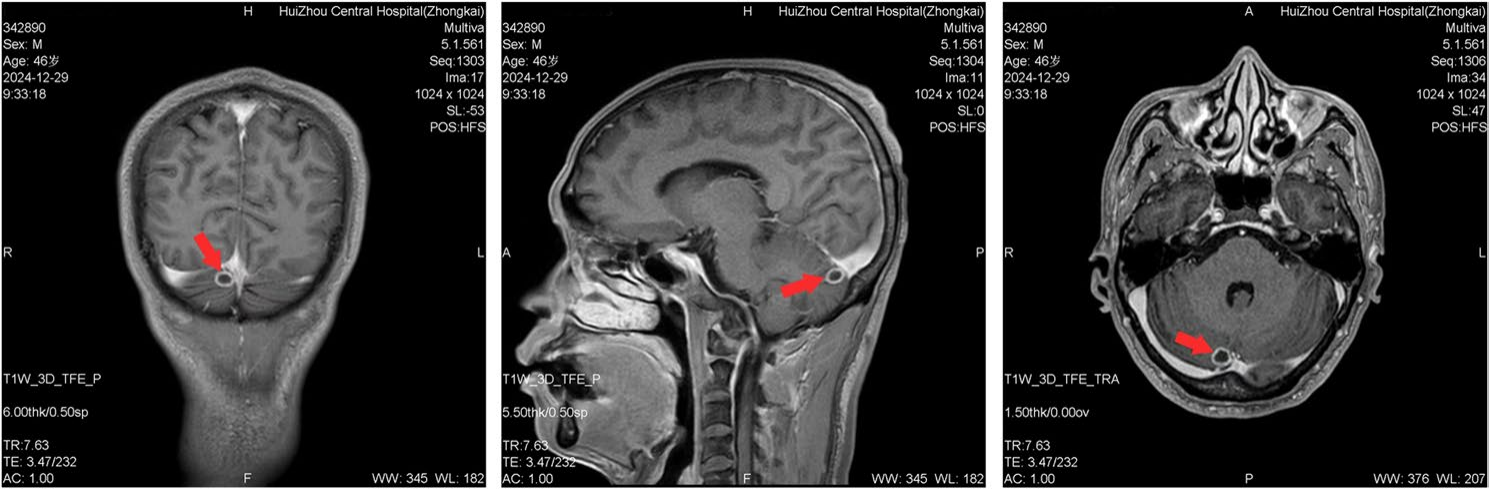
Brain MRI scans showed a 10-mm nodular lesion in the right cerebellar hemisphere (red arrow). *MRI* magnetic resonance imaging
